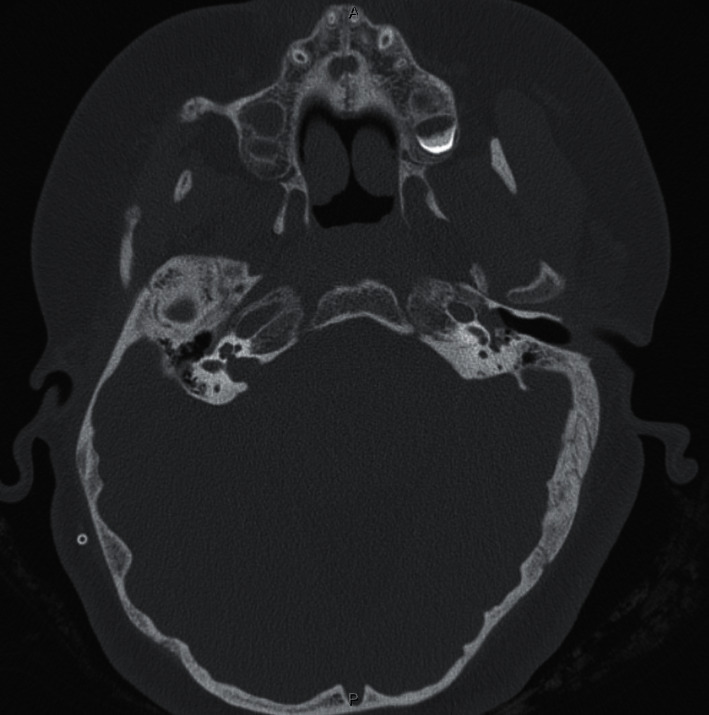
CT axial view. There is no visible stapes. There is no direct continuity between the mass and the carotid or jugular bulb.
